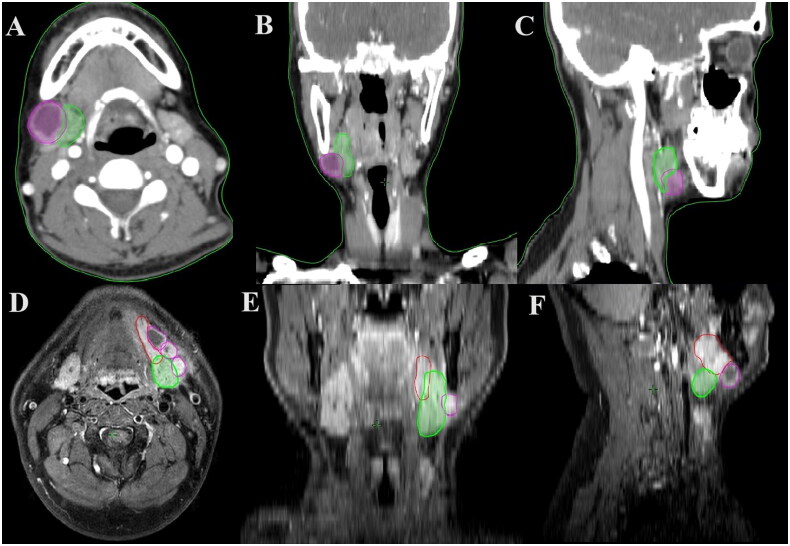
Two oral squamous cell carcinoma patients with preoperative imaging involving the submandibular gland (A-C: preoperative enhanced CT and postoperative pathology showed extra-capsular invasion of the submandibular gland by metastatic lymph nodes in the level IB in a patient with stage T1N3bM0 IVB squamous cell carcinoma of the right buccal mucosa [A: axial view, B: coronal view, C: sagittal view]. D-F: preoperative enhanced MRI and postoperative pathology showed invasion of the submandibular gland by metastatic lymph nodes in the level IB in a patient with stage T2N3bM0 IVB left tongue squamous cell carcinoma. The preoperative enhanced MRI showed indistinct boundaries between the primary tumor and the submandibular gland and sublingual gland in this patient [D: axial view, E: coronal view, F: sagittal view]). (red line, primary tumor; green line, submandibular gland; purple line, lymph node metastasis in level IB).
